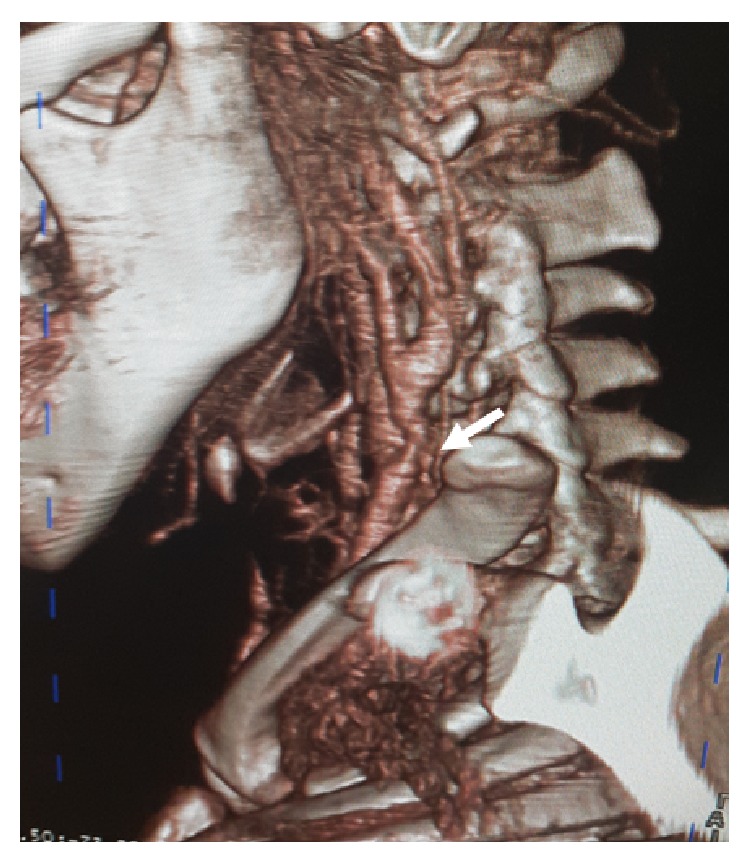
VRT CT picture at one-year follow-up. The common carotid is permeable without any residual dilation, with some mild scar narrowing at the anastomotic site (white arrow).
